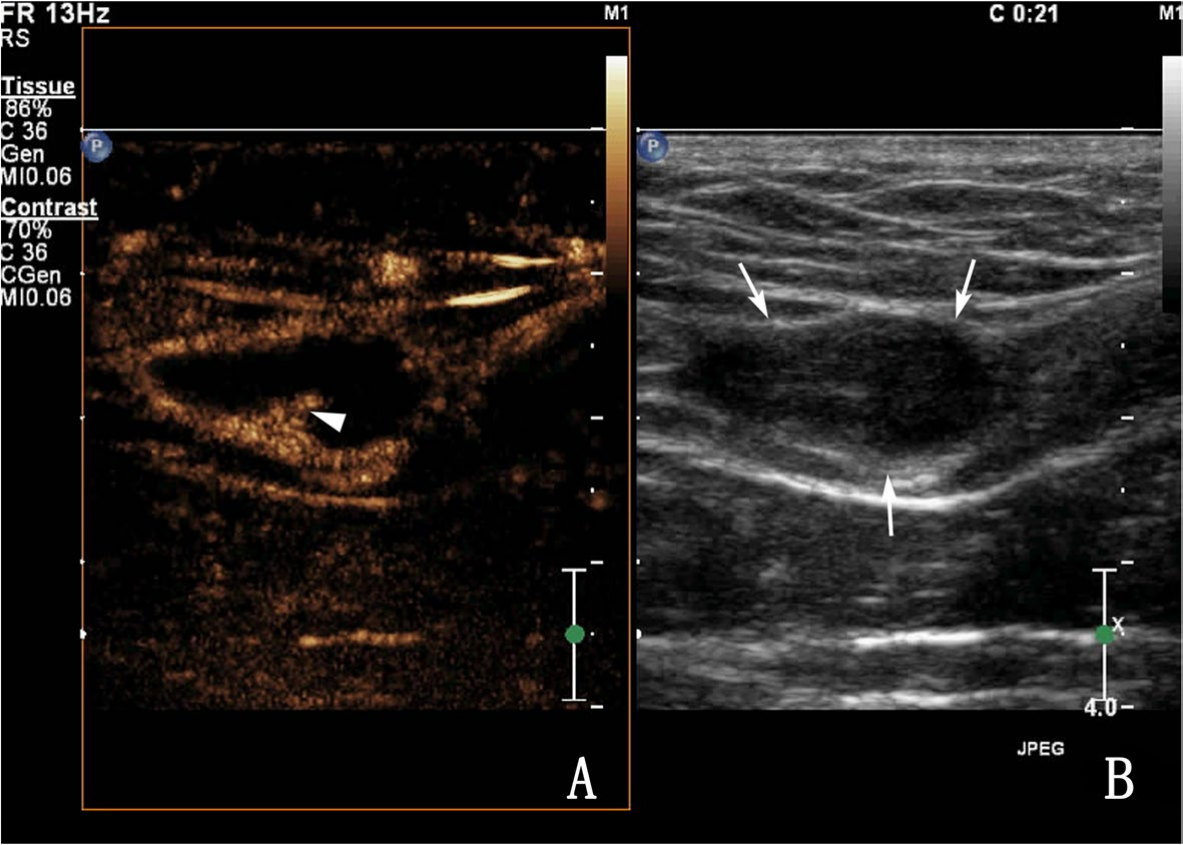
A 45-year-old male with tuberous vas deferens tuberculosis. A Routine ultrasonography showing a low-echo-type lesion (coarse arrow). B Contrast-enhanced ultrasonography showing heterogeneous enhancement with ring enhancement of a nodule (the small arrow shows a nodule-in-nodule) (Type IIc)
